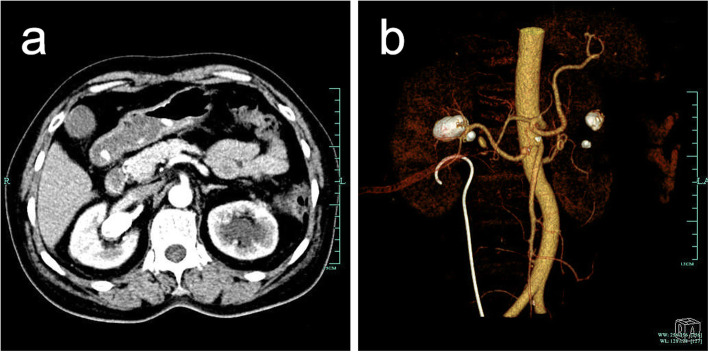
a The contrast-enhanced CT scan shows the right renal arterial branch penetrating into the aneurysm. b The coronal image and 3-dimensional reconstruction show multiple aneurysms in both kidneys. The large right RAA was located at the first bifurcation of the renal artery
